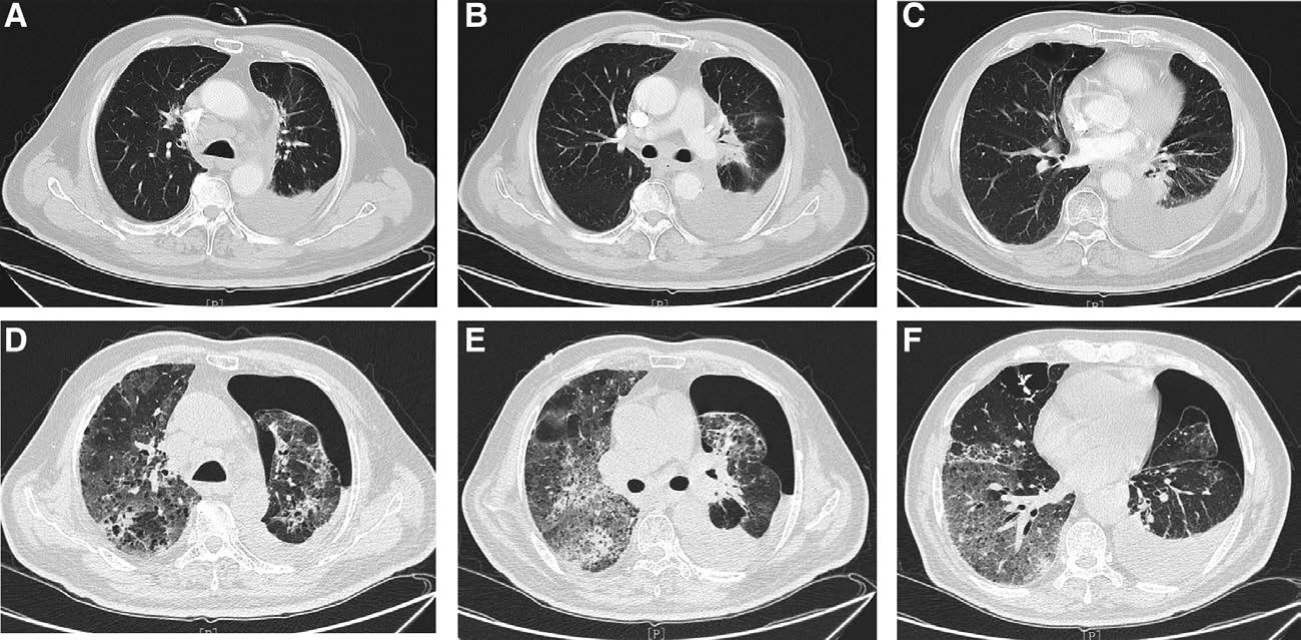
Immune-related pneumonitis. (A–C) After the fourth course of chemotherapy + durvalumab treatment, a chest CT scan showed no obvious inflammatory lesions in the lungs. (D–F) Chest CT scan on admission (5 weeks after the eighth course of chemotherapy + durvalumab treatment) showed non-segmentally distributed extensive consolidations and GGOs in the bilateral lungs. CT = computed tomography, GGOs = ground-glass opacities.
